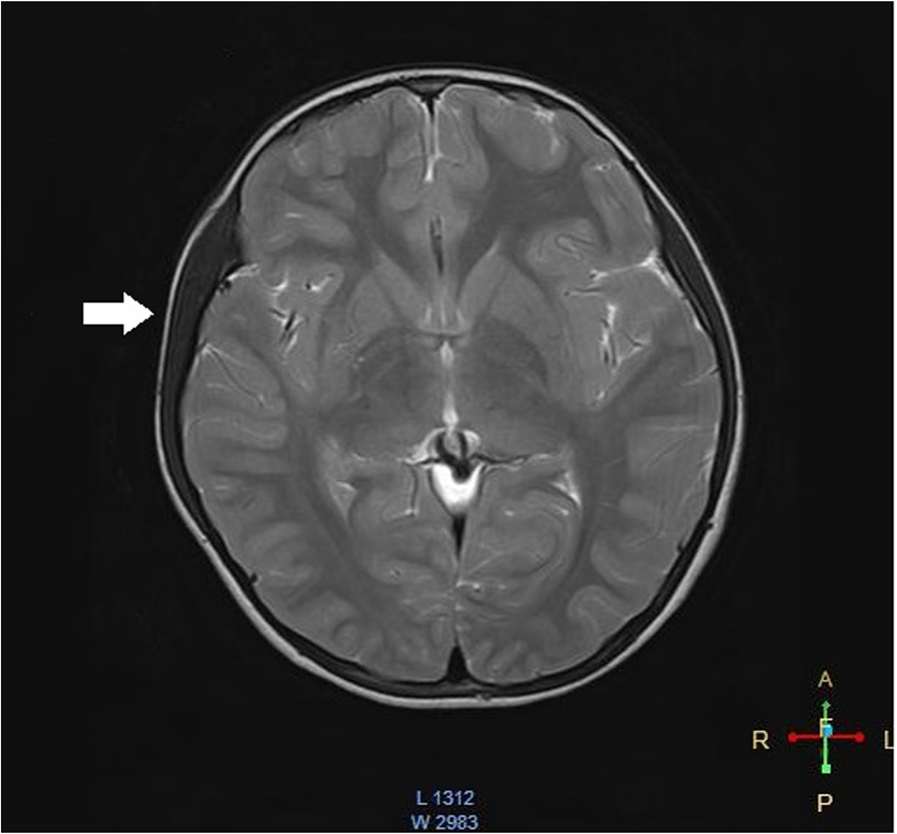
Isolated right temporalis muscle hypertrophy on an axial MRI image. T2W image of the cMRI study demonstrating enlarged right temporalis muscle on an axial section without abnormal contrast enhancement. White arrow head indicate enlarged right temporalis muscle
